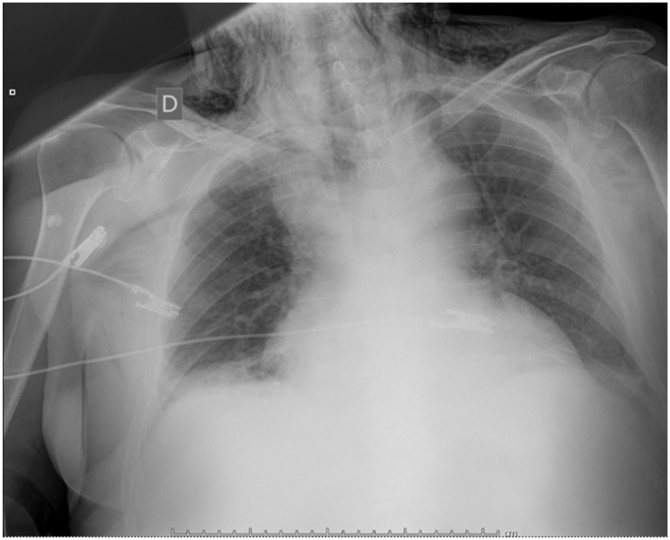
AP chest x-ray shows widened mediastinum.
